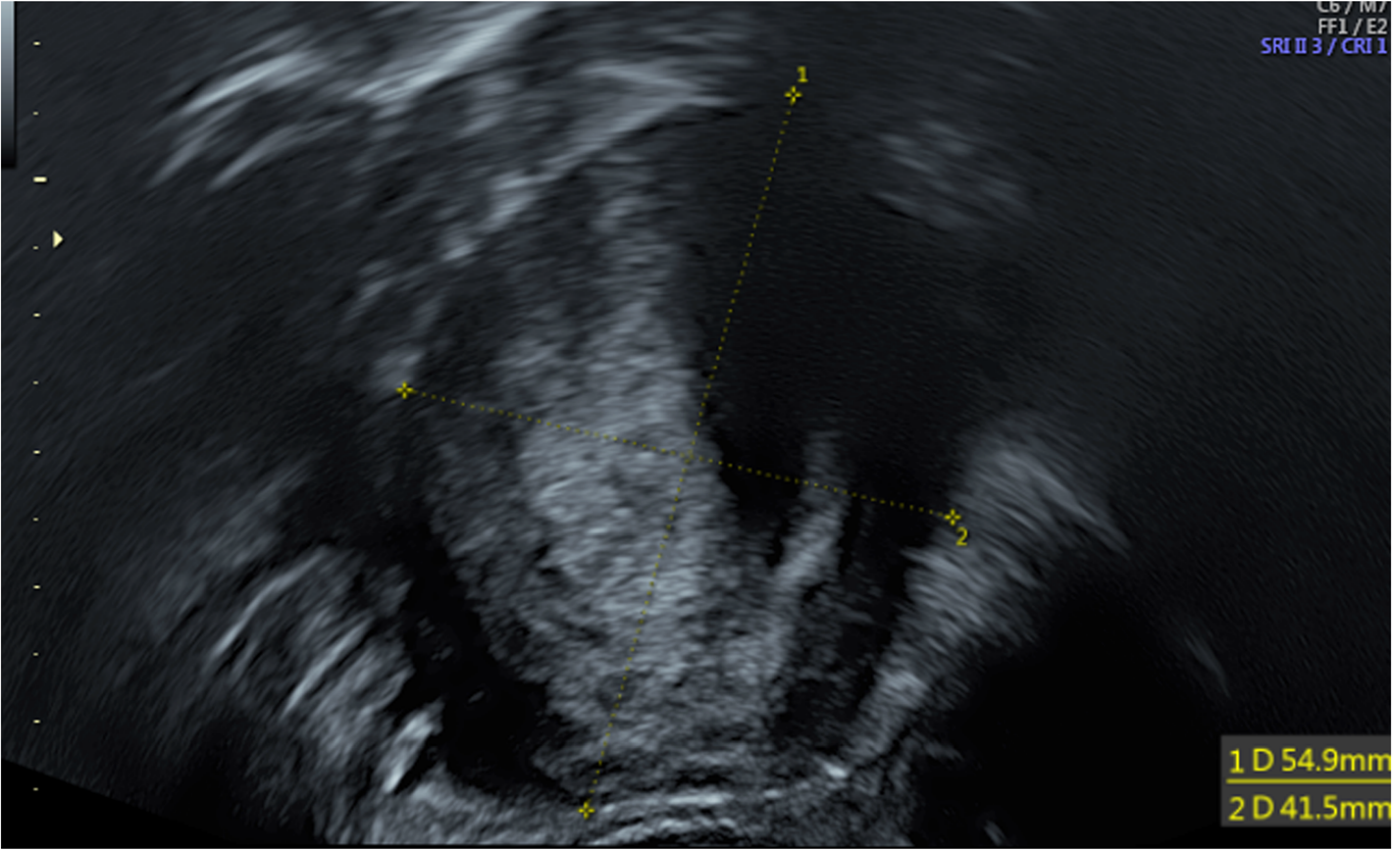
Transvaginal ultrasound revealed heterogenic pelvic mass and acoustic shadows typical for leiomyoma. (Voluson S10, GE Health, Chicago, Illinois, USA)
